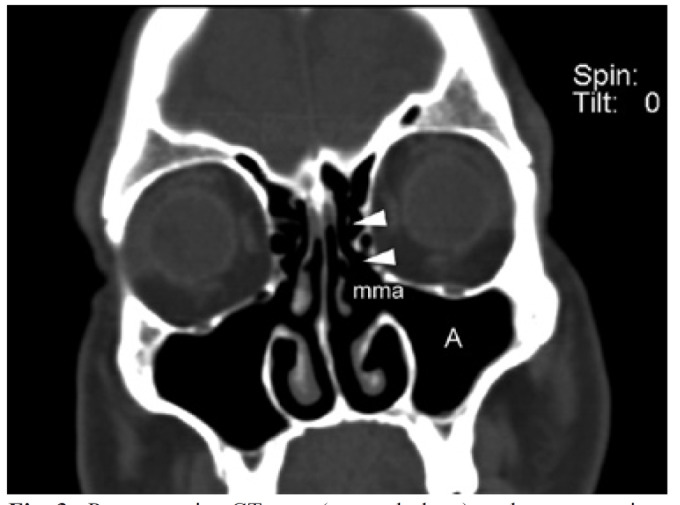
Postoperative CT scan (coronal plane) to the same patient of figure 2. The maxillary sinus (A) and the anterior ethmoid (arrowheads) are perfectly ventilated trough the middle meatal antrostomy (mma) associated to anterior ethmoidectomy, made by functional endoscopy sinus surgery.
